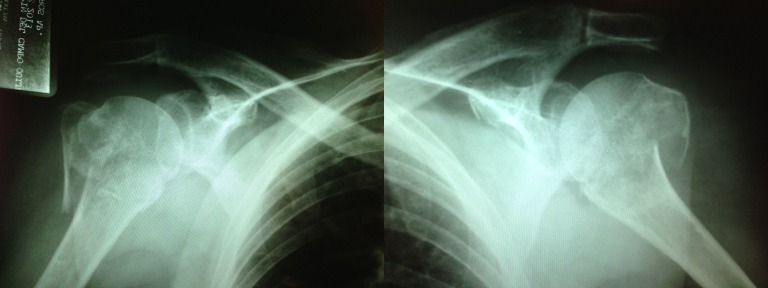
Simple x-ray AP bilateral views
